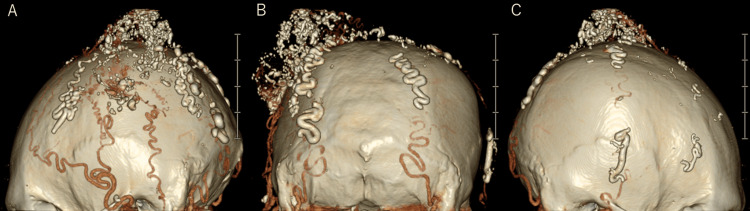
Post-embolization 3DCTA (A: left lateral view, B: posterior view, C: right lateral view) Post-embolization 3DCTA shows NBCA filling within the feeding arteries and the nidus. 3DCTA: three-dimensional computed tomography angiography, NBCA: n-butyl-2-cyanoacrylate
